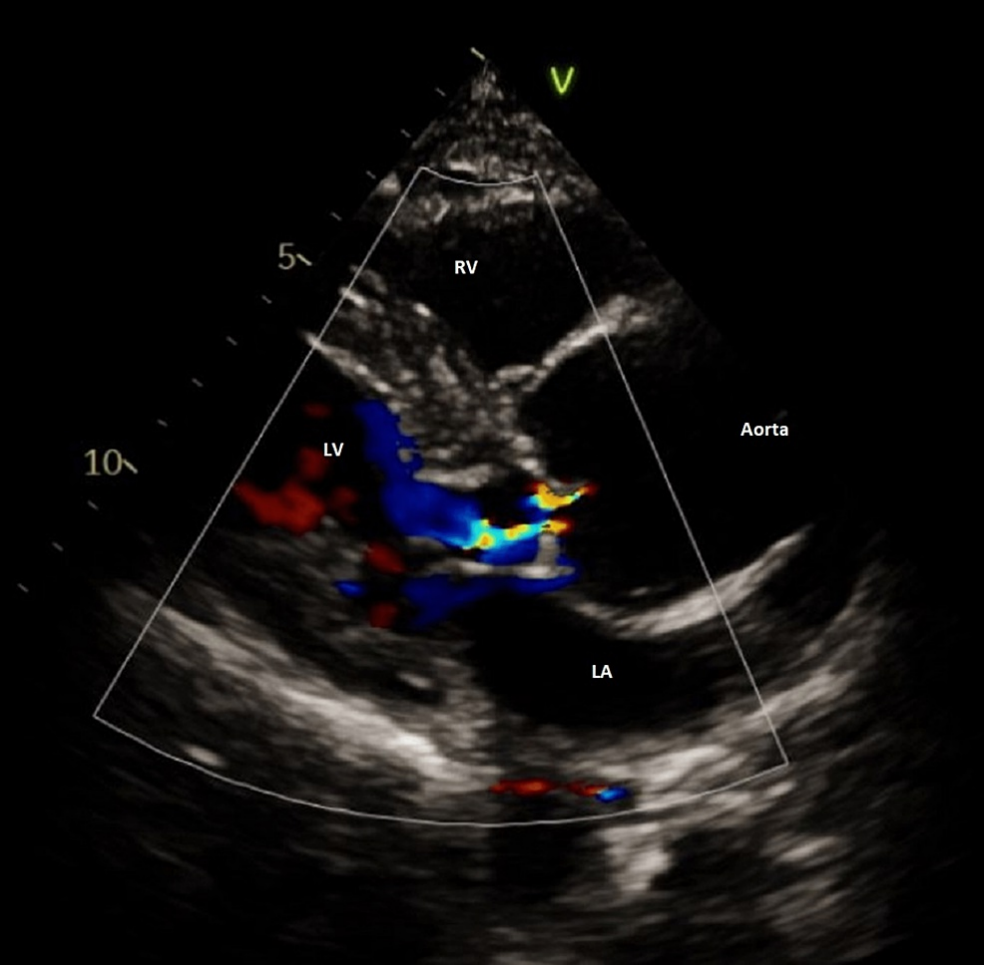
Echocardiogram revealing moderate aortic regurgitation and mitral regurgitation LA: Left Atrium; LV: Left Ventricle; RV: Right Ventricle
